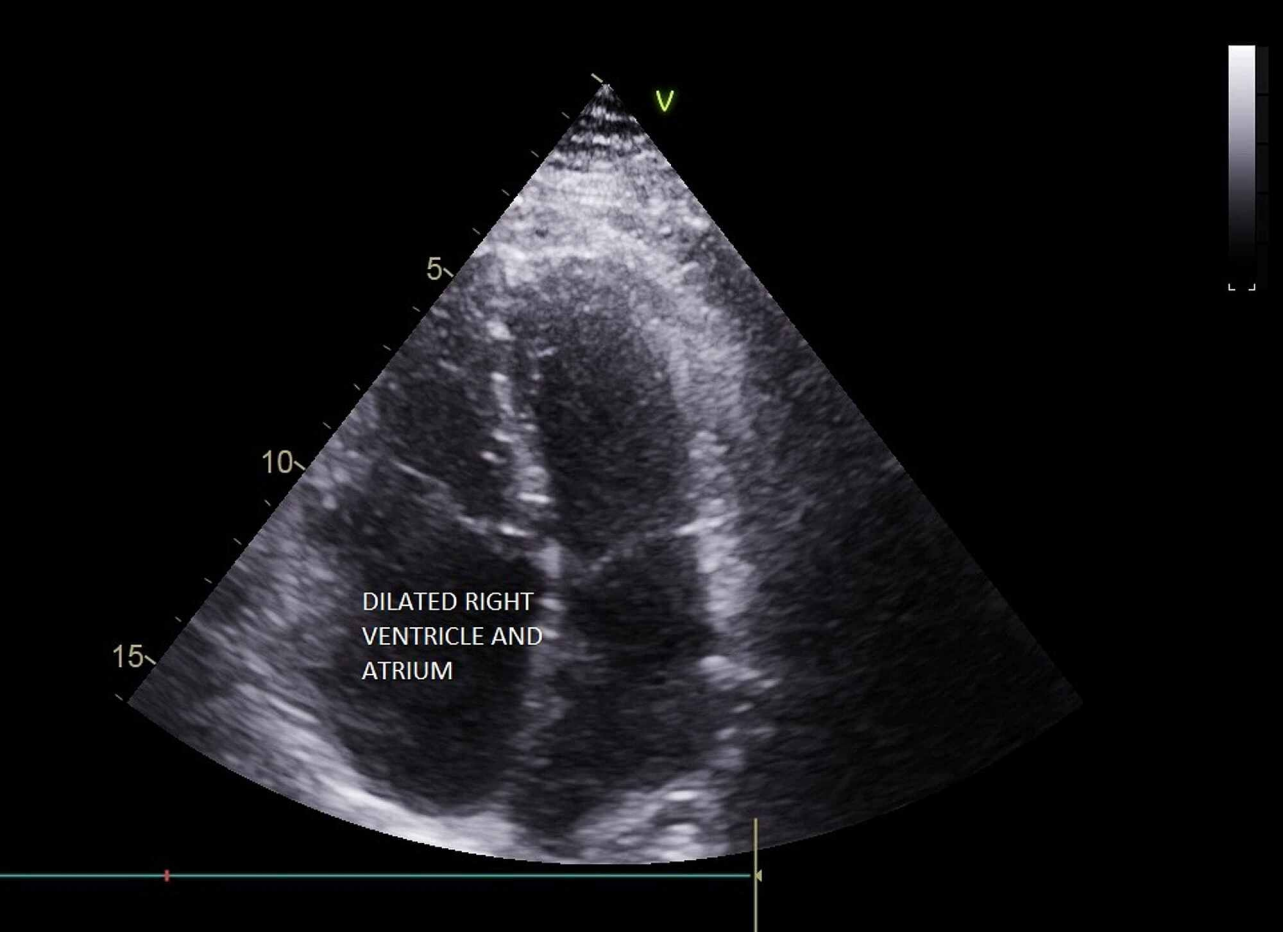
Apical four-chamber view showing dilation of the right atrium and ventricle
